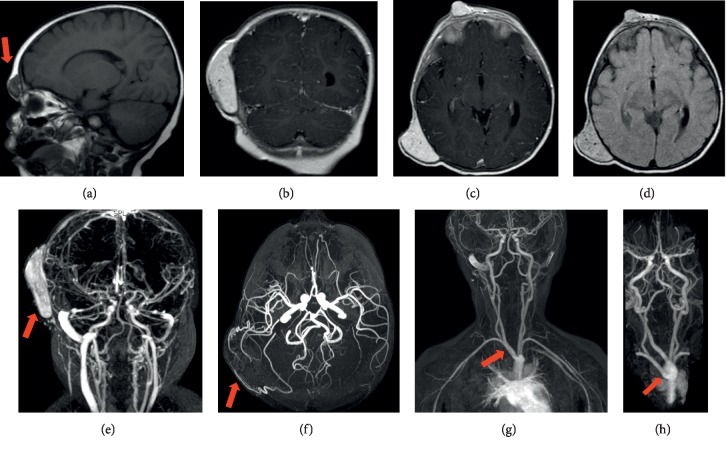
MRI/MRA images (case 2). (a) Sagittal MRI (T1) image of the brain showing a 1.2 cm diameter extracranial hemangioma on the right frontal scalp (red arrow). (b) Coronal MRI (postcontrast T1W) image showing the larger hemangioma on the right parietal scalp. (c) Axial MRI image (postcontrast T1W) showing both the smaller hemangioma on the right frontal scalp and the larger hemangioma on the right parietal scalp measured 2.9 × 2.3 cm axially. (d) Axial MRI image (SWI) showing both the smaller hemangioma on the right frontal scalp and the larger hemangioma on the right parietal scalp. (e) Head and neck MRA showing intracranial circulation and the large right parietal scalp hemangioma (red arrow). (f) Head MRA showing intracranial circulation and direct circulation (red arrow) to the right parietal scalp hemangioma. (g) Upper body MRA showing branching of upper body large arteries. (h) Zoomed in and angled upper body MRA showing the aberrant right subclavian artery (red arrow) coming directly off the aortic arch and not from the brachiocephalic artery (as it normally should). There is no observed right brachiocephalic artery.
